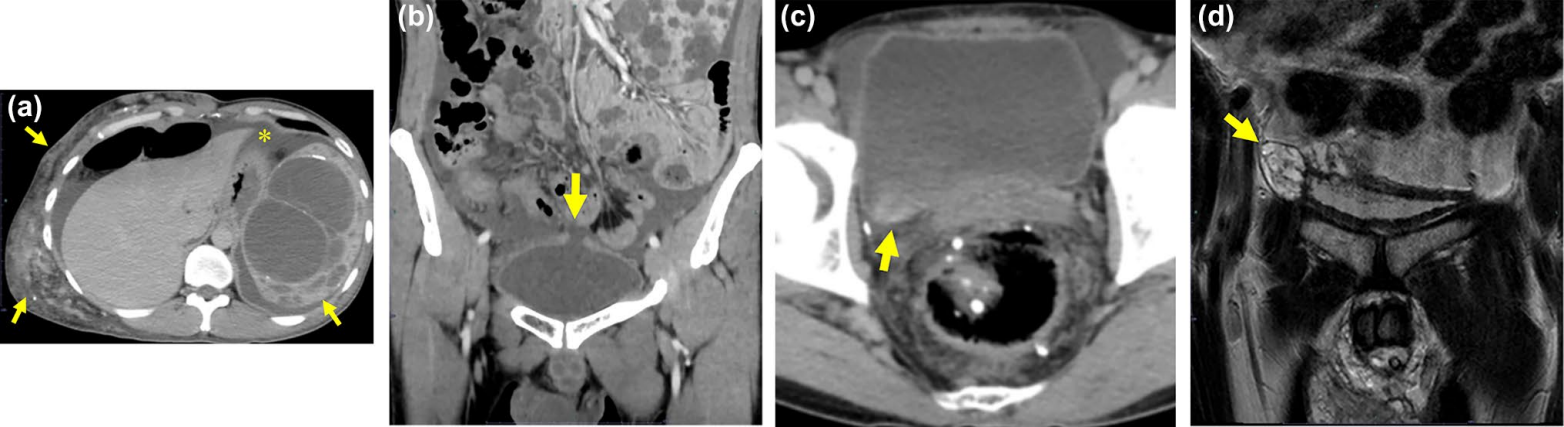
40-Year-old male with bladder rupture due to bladder hemangioma associated with Klippel-Trenaunay-Weber syndrome. He presented with lower abdominal pain and pseudo-renal failure. a CECT shows massive ascites (*) and lymphangioma of subcutaneous and spleen (arrows) associated with Klippel–Trenaunay–Weber syndrome. b The coronal image shows the discontinuity of bladder wall (arrow) and low dense uremic ascites. c Small amount of contrast medium has reached to the bladder (arrow) in delayed phase (180 s after administration of contrast medium) but the uremic ascites showed still low density (*). It is difficult to identify the leakage of contrast medium. d T2-weighted MR image shows bladder hemangioma as the cause of bladder hemorrhagic tamponade in the past (arrow). The fragility of the bladder wall may be caused by the history of tamponade and several placements of catheter
